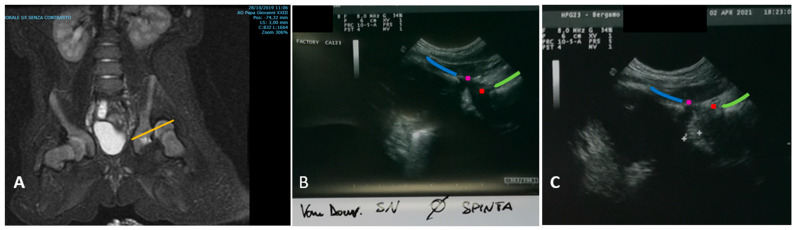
(A) MR image (coronal plane) of a dislocated hip (patient Id Nr 4, Graf type IV, see text). The yellow line indicates the plane in which the hips are scanned with the miniconvex probe, using TIT technique. Looking at this image, it is easier to understand why the anatomical landmarks indicated in Figure 2 (ramus superior os pubis, labrum, femoral head and neck) must all be present in the ultrasound images obtained with TIT. Particularly, if the labrum is missing, the femoral head could be dislocated proximally and the iliac wing wrongly identified as os pubis, obtaining a false negative. (B) Another patient with a dislocated hip (Graf type IV) examined with TIT. The anatomical landmarks are indicated with the same colors as Figure 2B. The anterior contour of the ramus superior os pubis is marked with a blue line, the anterior border of the labrum is marked with a violet point. The anterior border of the head is marked with a red point and the anterior contour of the neck of the femur is marked with a green line. Here, the hip is dislocated, and the imaginary extension of the lines built by the anterior margin of pubis, labrum, femoral head and neck lies on different curves. (C) The same hip from (B), after reduction. The hip is now reduced, and the imaginary extension of the lines built by the anterior margin of pubis, labrum, femoral head and neck lies on the same curve.
